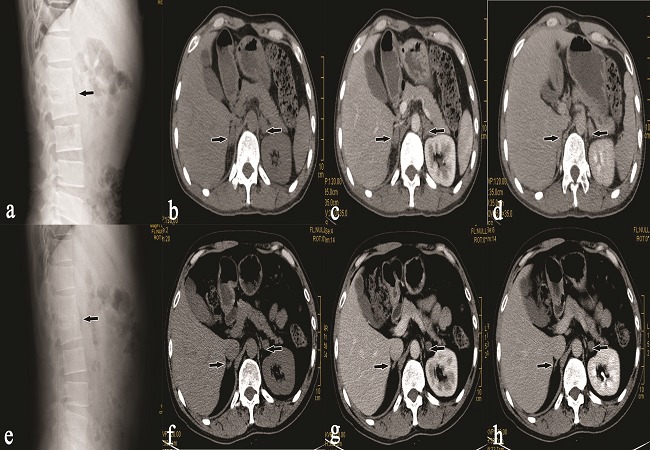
Pre-treatment (a) and post treatment (e) lateral lumbar plain film showing fusion and irregular osteolytic destruction of the L1, L2 vertebral bodies (arrow). Pre-treatment (b, c, d) and Post-treatment (f, g, h) CT- abdomen of different phase showing bilateral enlargement of adrenal gland (upper arrow) with irregular enhancement and lesion like changes and decrease in adrenal gland size (lower arrow) and significant reduction in adrenal lesions respectively
